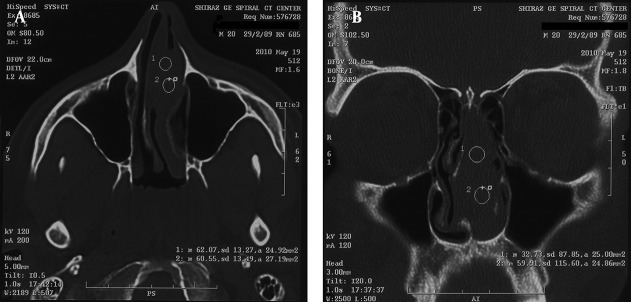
(A, B). CT Scan-Axial View, Left Nasal Cavity Mass with Septal Deviation
